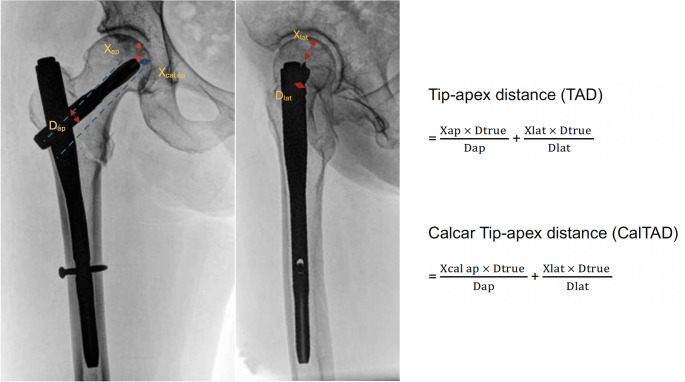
Tip-apex distance (1) and calcar tip-apex distance (CalTAD) measurement on anteroposterior and lateral radiographs. Both are the sums of distance from helical blade tip to the apex of femoral head using femoral neck axis (Xap) or to the line parallel and adjacent to medical cortex (Xcal ap) in anteroposterior view and lateral view (Xlat), and having correction for magnification by the ratio of true nail diameter (Dtrue) and measured nail diameter (Dap or Dlat)
